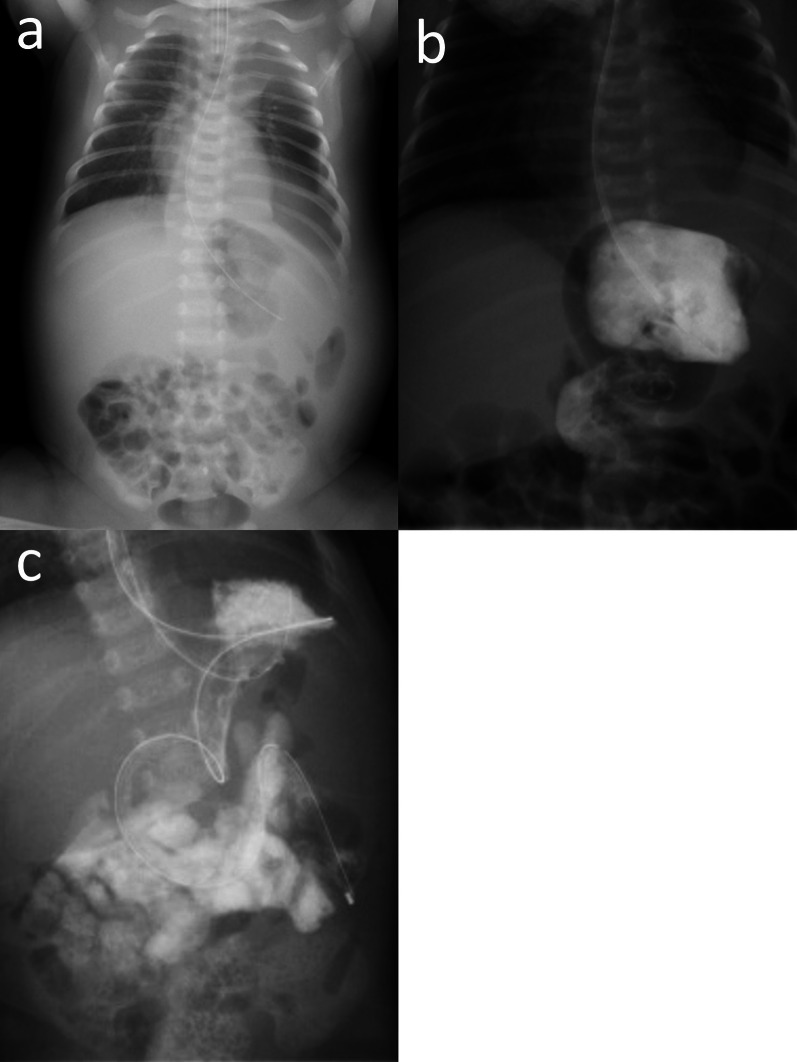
Abdominal X-ray (a) and upper gastrointestinal series (b, c). a Abdominal X-ray on postoperative day 1 demonstrated that the left diaphragm was pulled caudally, and the stomach was deviated medially. b Upper gastrointestinal series showed organo-axial gastric volvulus with the greater curvature crossing the stomach anteriorly. c The patient presented with recurrent gastric volvulus. In addition to gastric decompression, an enteral feeding tube was placed in the duodenum, and enteral feeding through the tube was initiated
